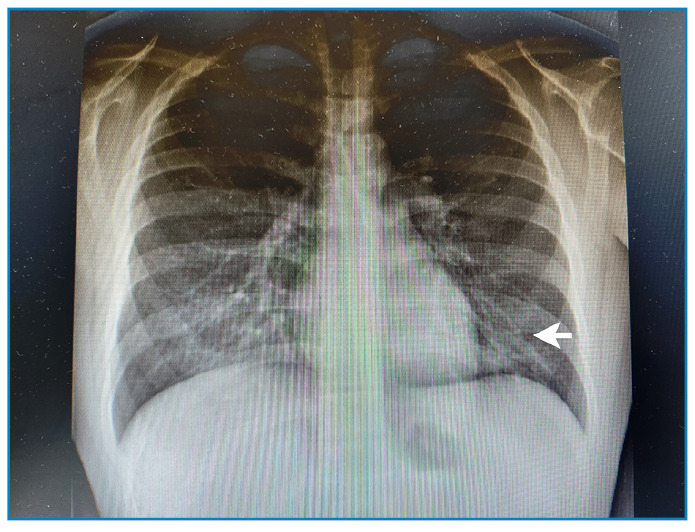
Chest radiograph: Opacity in the perihilar left field showed by the arrow.
